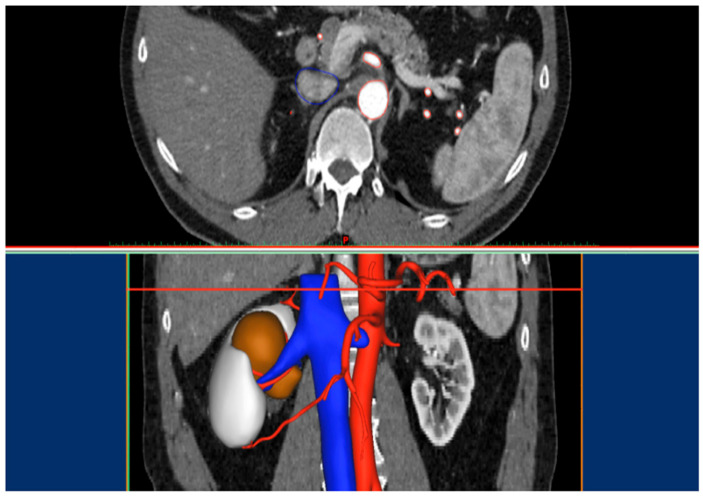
CT-based vascular segmentation (upper section) and subsequent three-dimensional volumetric reconstruction (lower section).
